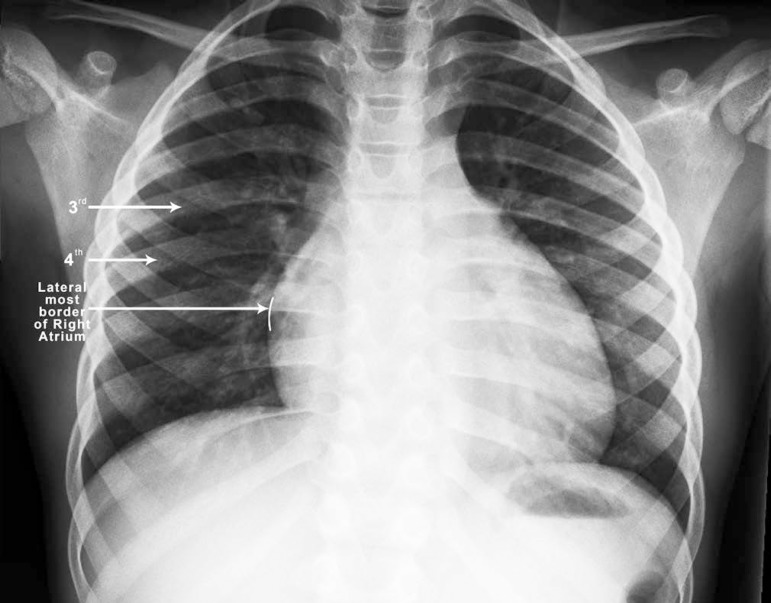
Chest X-ray with posteroanterior (PA) view showing the lateral
